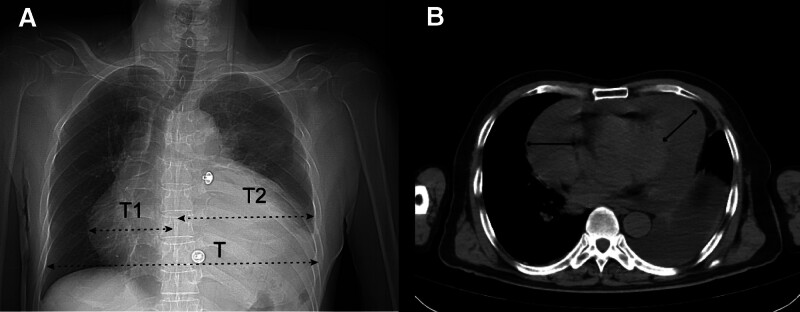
CT scan image on the 10th d after receiving Endostar treatment. (A) The cardiothoracic ratio was significantly larger than before treatment, exceeding 0.6. Cardiothoracic ratio = (T1 + T2)/T, where T1 represents the distance from the left heart margin to the midline, T2 represents the distance from the right heart margin to the midline, and T represents the maximum transverse diameter passing through the highest point of the right diaphragm. (B) A plain CT scan revealed acute pericardial tamponade and massive pericardial effusion, which were significantly increased compared to before the administration of Endostar. CT = computed tomography.
